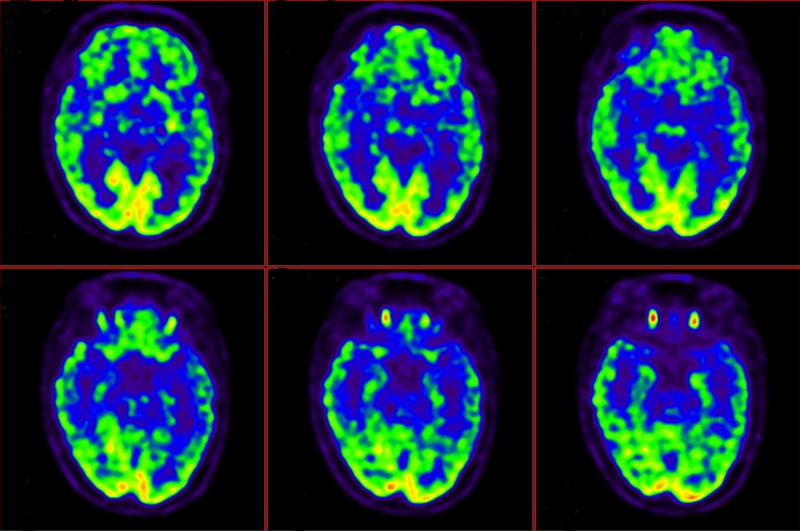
PET-CT of patient’s brain.
